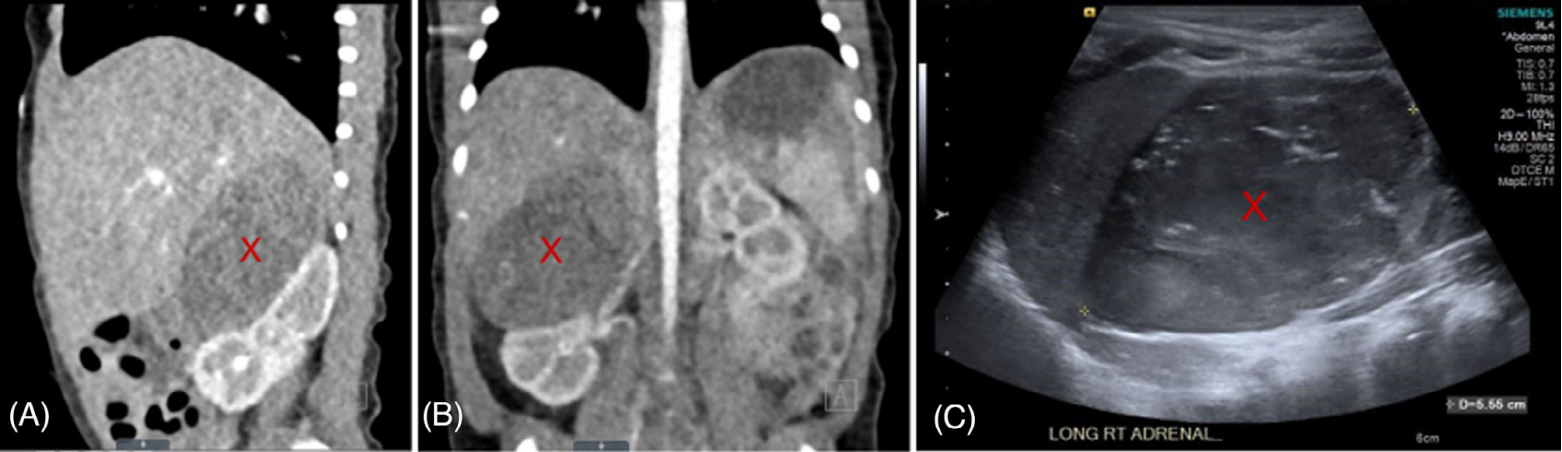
Right adrenal mass (marked with X) shown on initial sagittal CT (A), coronal CT (B), and follow‐up ultrasound (C).
